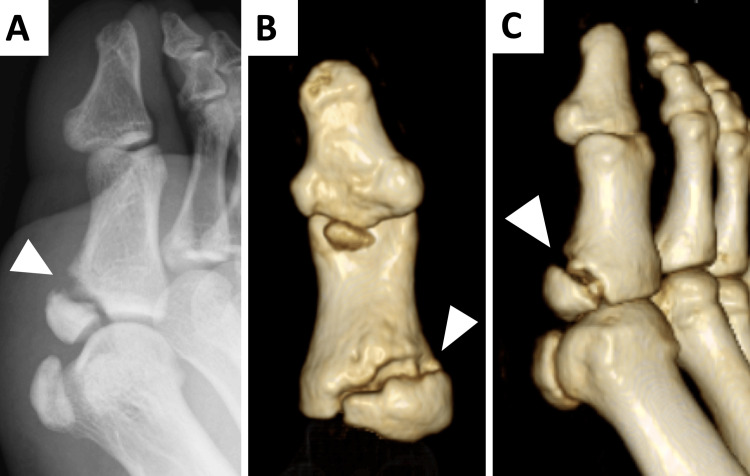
Radiography and CT showed the dislocation of the non-union area of the proximal phalanx of the great toe A: oblique view on X-ray; B: plantar side of the great toe on 3D CT; C: medial side of the great toe on 3D CT 3D: three dimensional, CT: computed tomography
